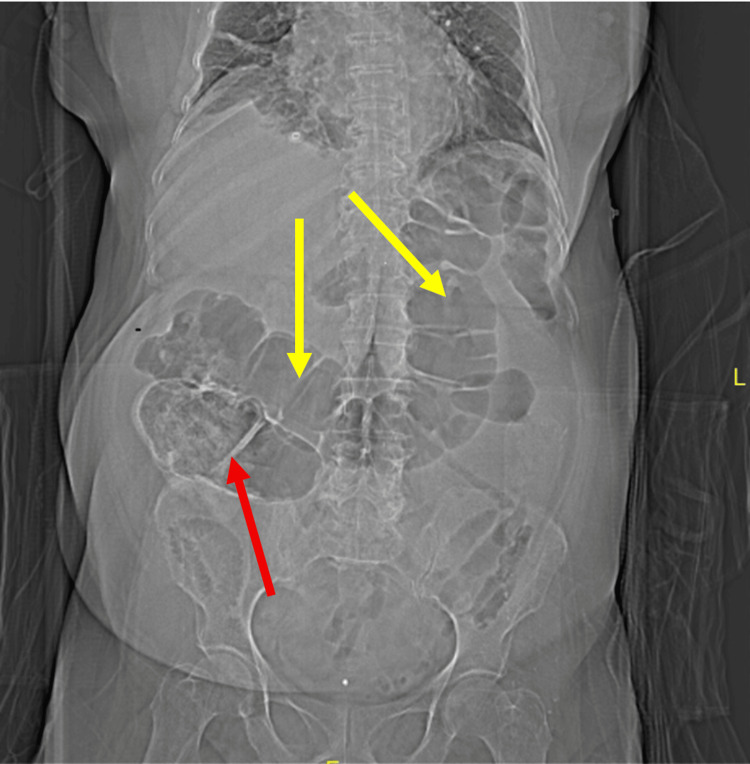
Contrast-enhanced CT abdomen showing anterior displacement and distension of the cecum. Preoperative abdominal radiograph demonstrating a low-mechanical large bowel obstruction with a massively distended, gas-filled cecal loop displaced into the central abdomen (red arrow). The yellow arrow highlights the pronounced distension of the ascending colon, confirming significant proximal large bowel dilation.
